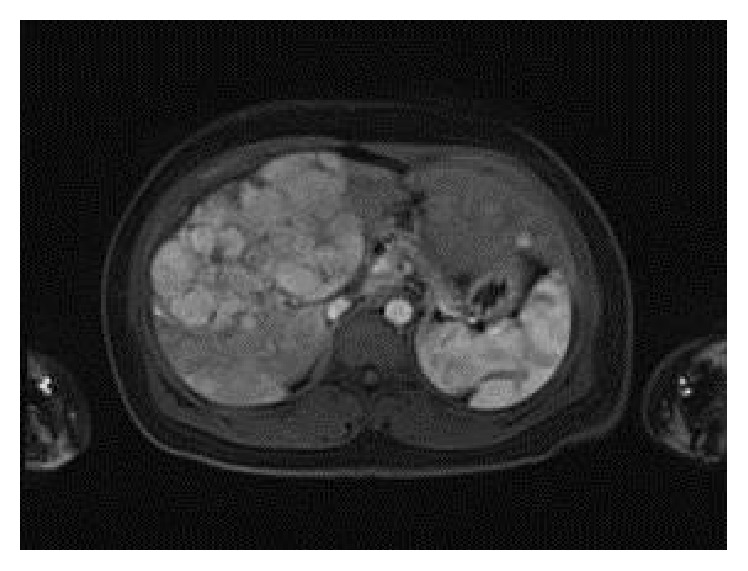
MRI of the abdomen with contrast shows multiple lesions in the right and left liver lobes, the largest 8.5 cm.
